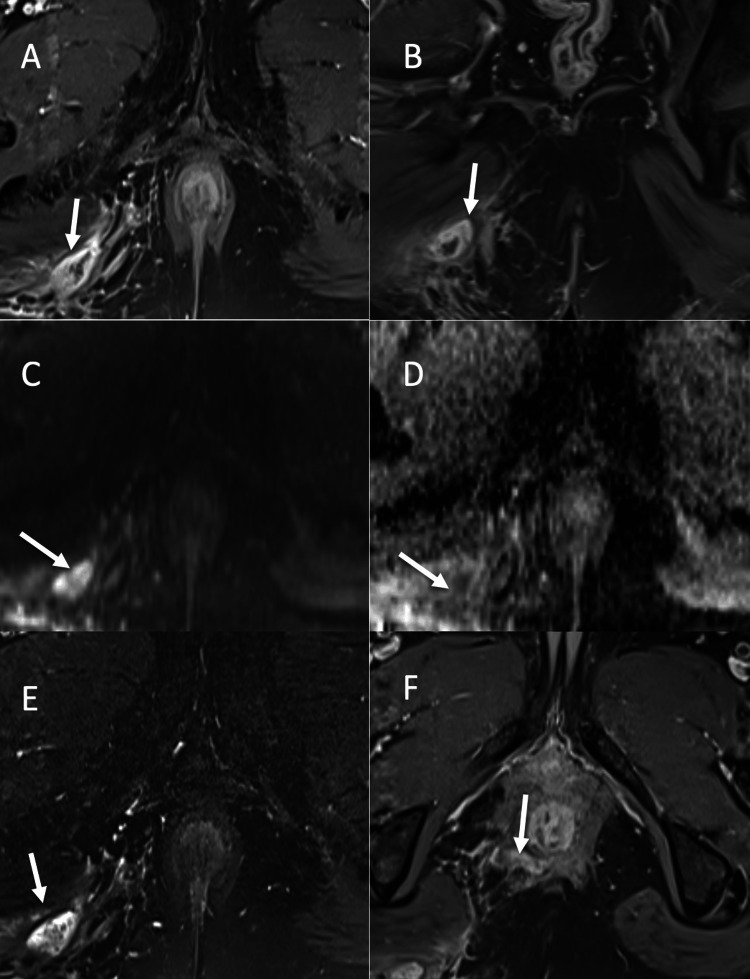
Perianal abscess and inflammation in grade 4 trans-sphincteric fistula: (A-B) Axial and coronal post contrast fat suppressed T1-WI respectively, showing heterogeneously and predominantly peripherally enhancing abscess collection in right perianal region. (C-E) Axial DWI, ADC and STIR respectively, depicting abscess showing hyperintense signal on DWI (C) with corresponding signal drop on ADC (D) and hyperintense signal on T2 STIR (E). Note the perianal inflammation showing hyperintense signal on T2 STIR with not restriction on DWI. (F) Axial post contrast fat suppressed T1-WI showing a curvilinear trans-sphincteric fistula coursing the external sphincter at 8-o’clock position. T1-WI: T1 weighted image; DWI: diffusion weighted imaging, ADC: apparent diffusion coefficient; STIR: short tau inversion recovery
